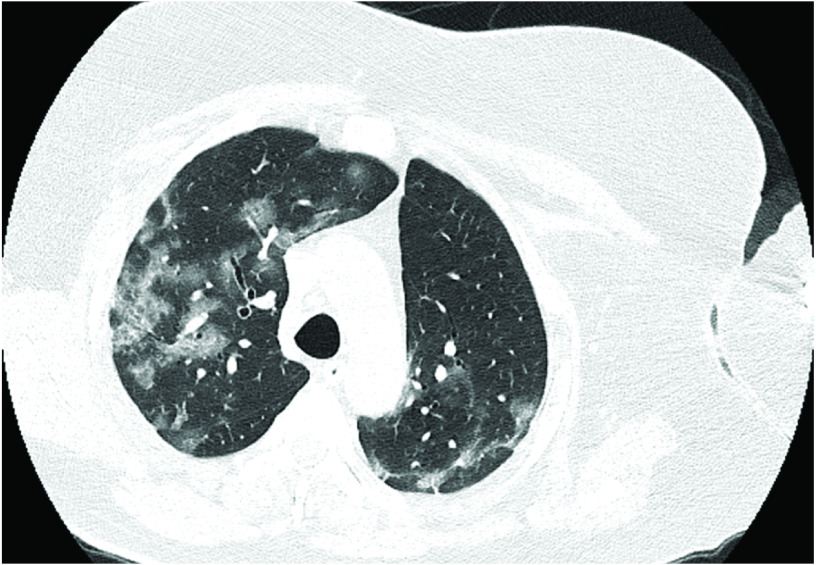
High-resolution CT scan lung showing ground glass opacities in a COVID-positive male patient.
